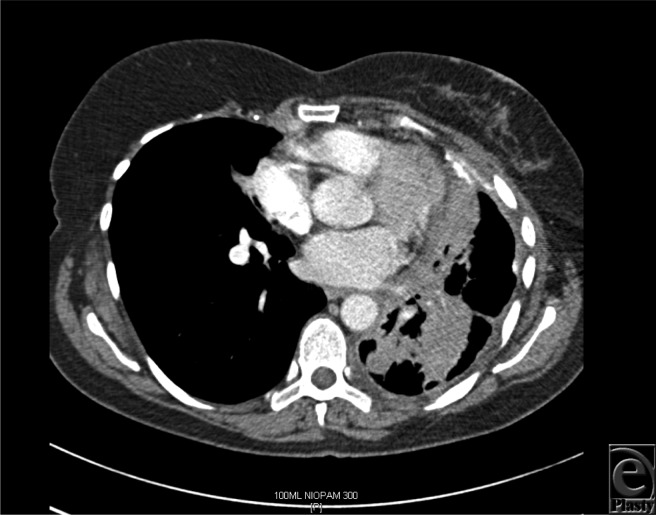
Computed tomographic scan of patient A demonstrating intrapleural metastases, intrapulmonary nodes, and thickening of the left pleural surface.
